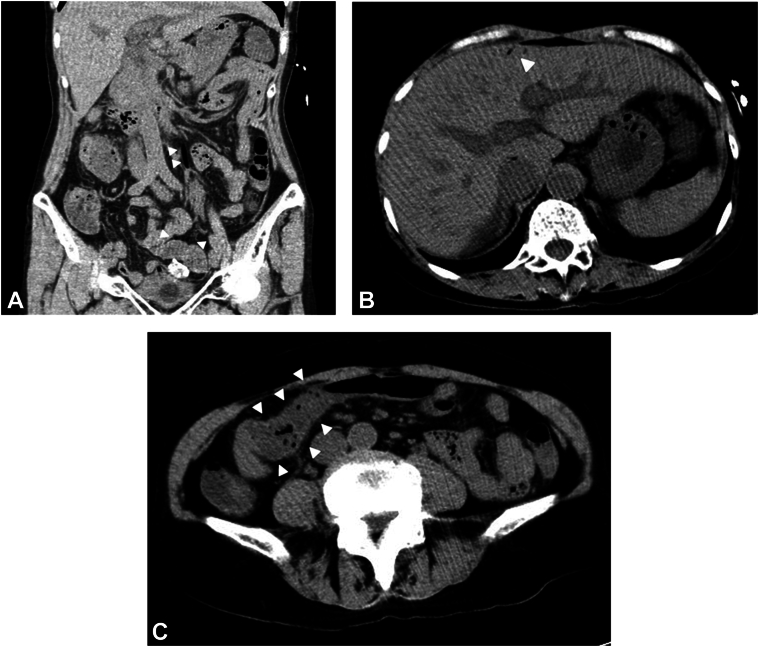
A and B, CT imaging 5 hours after endoscopic submucosal dissection revealed gas in the peripheral branches of the liver and the mesenteric veins (*white arrows*), indicating hepatic portal venous gas extending throughout the superior and inferior mesenteric vein. C, The transverse colon was edematous, but no free air or ascites was observed.
